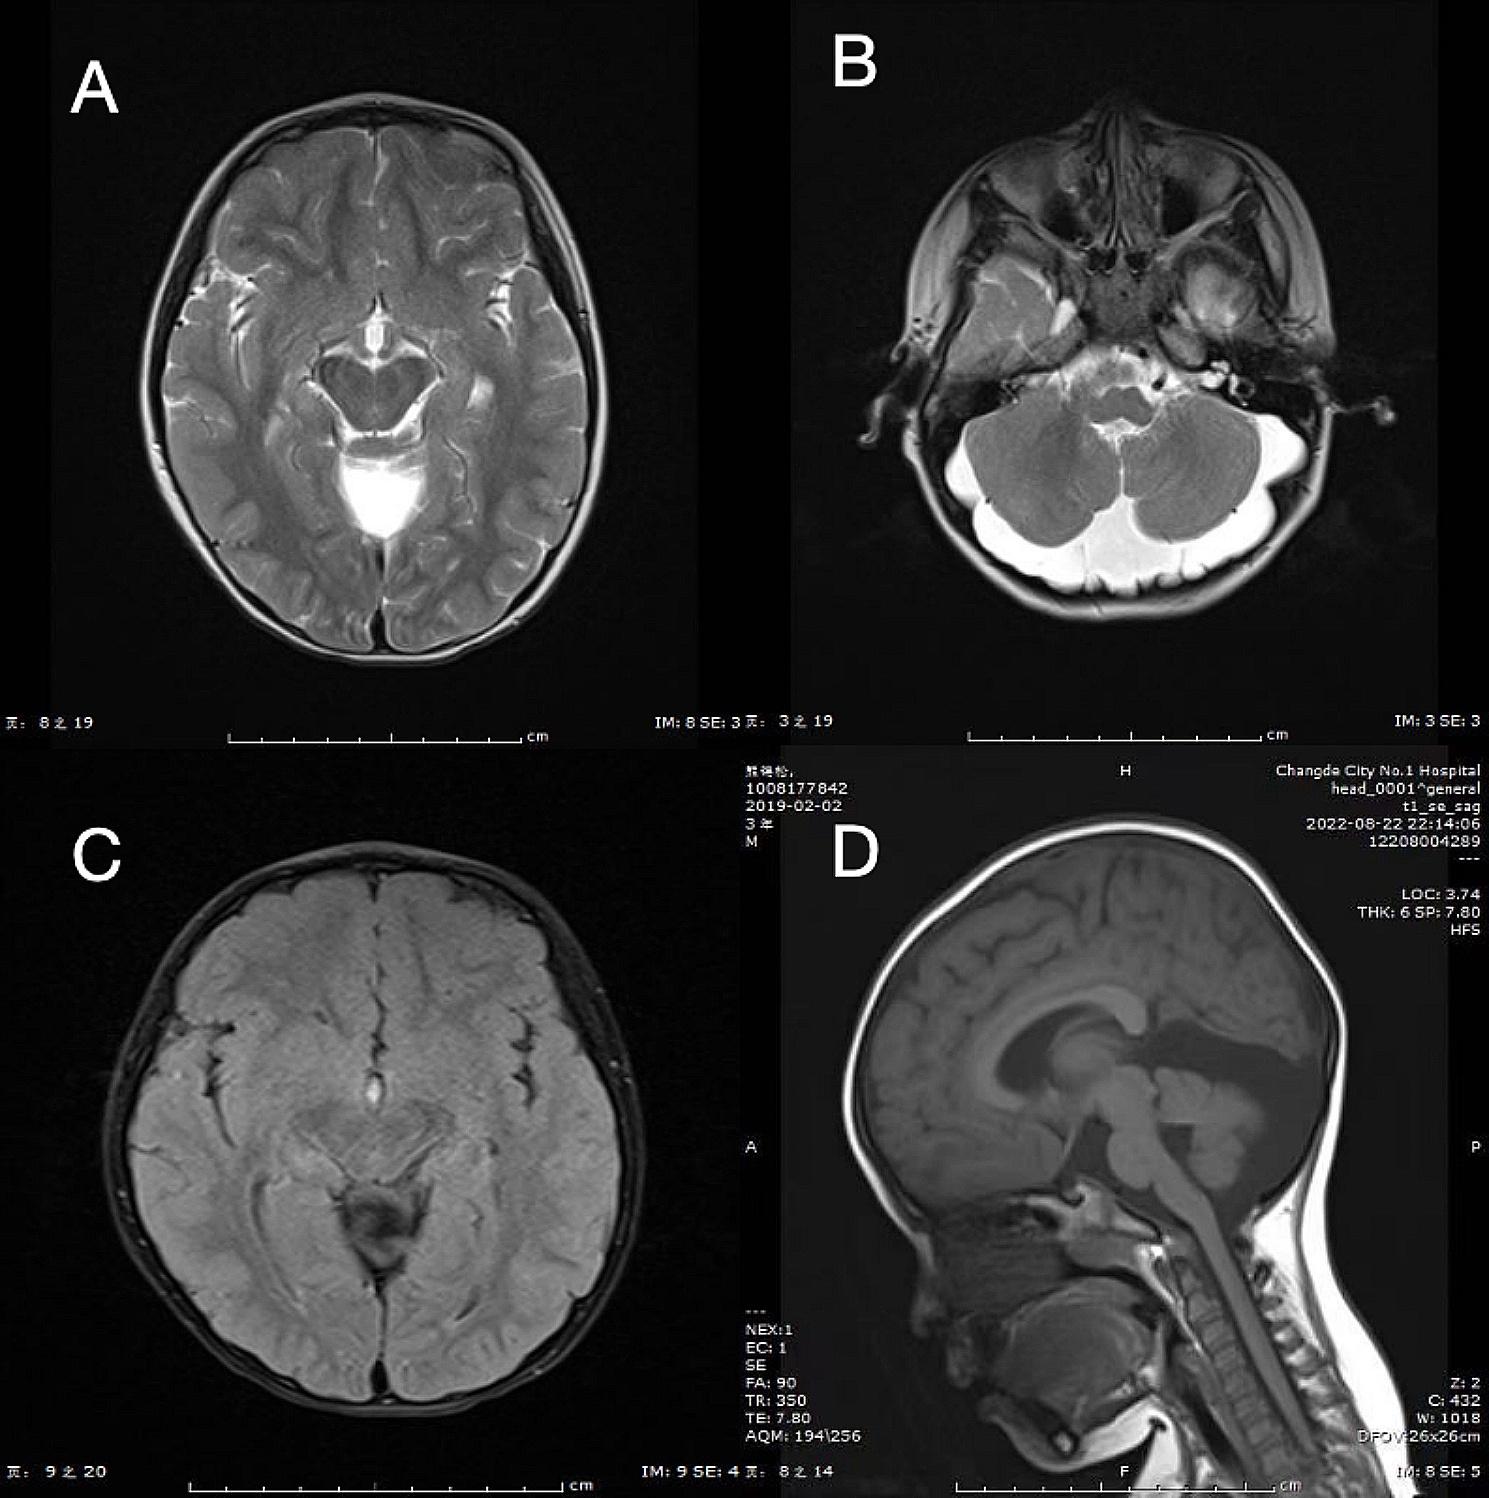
axi T2W1 (A), axi T2W1 (B), axi T2FLAIR v (C), and sag T1W1 (D) magnetic resonance images of the proband. Note: Bilateral cerebellar hemispheres are small, cerebellar vermis is asymmetrical, cisterna occipitalis, cisterna quadrimata and ventricles are enlarged, bilateral ventricles are slightly widened, hydrazine bodies are thin, midline is centered, bone and soft tissue are not significantly abnormal. Diagnosis: cerebellar vermis changes, abnormal development? Hydrocephalus
